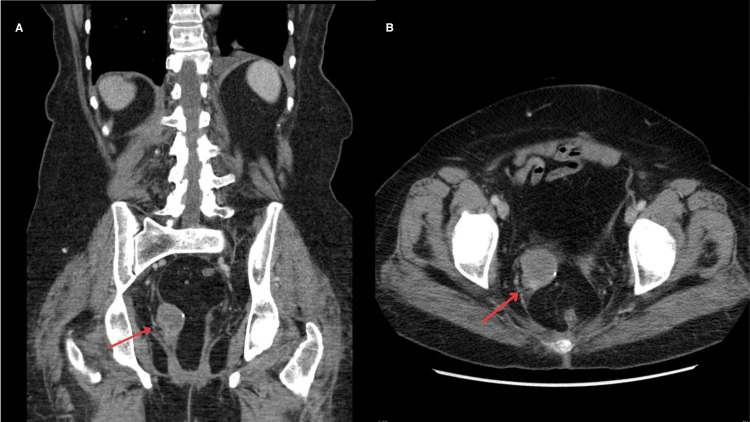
CT scans WOC with tumor at the vaginal cuff Coronal (A) and axial (B) CT scans WOC taken in 2020 showing a new tumor at the vaginal cuff (red arrows). CT: computed tomography; WOC: without contrast
